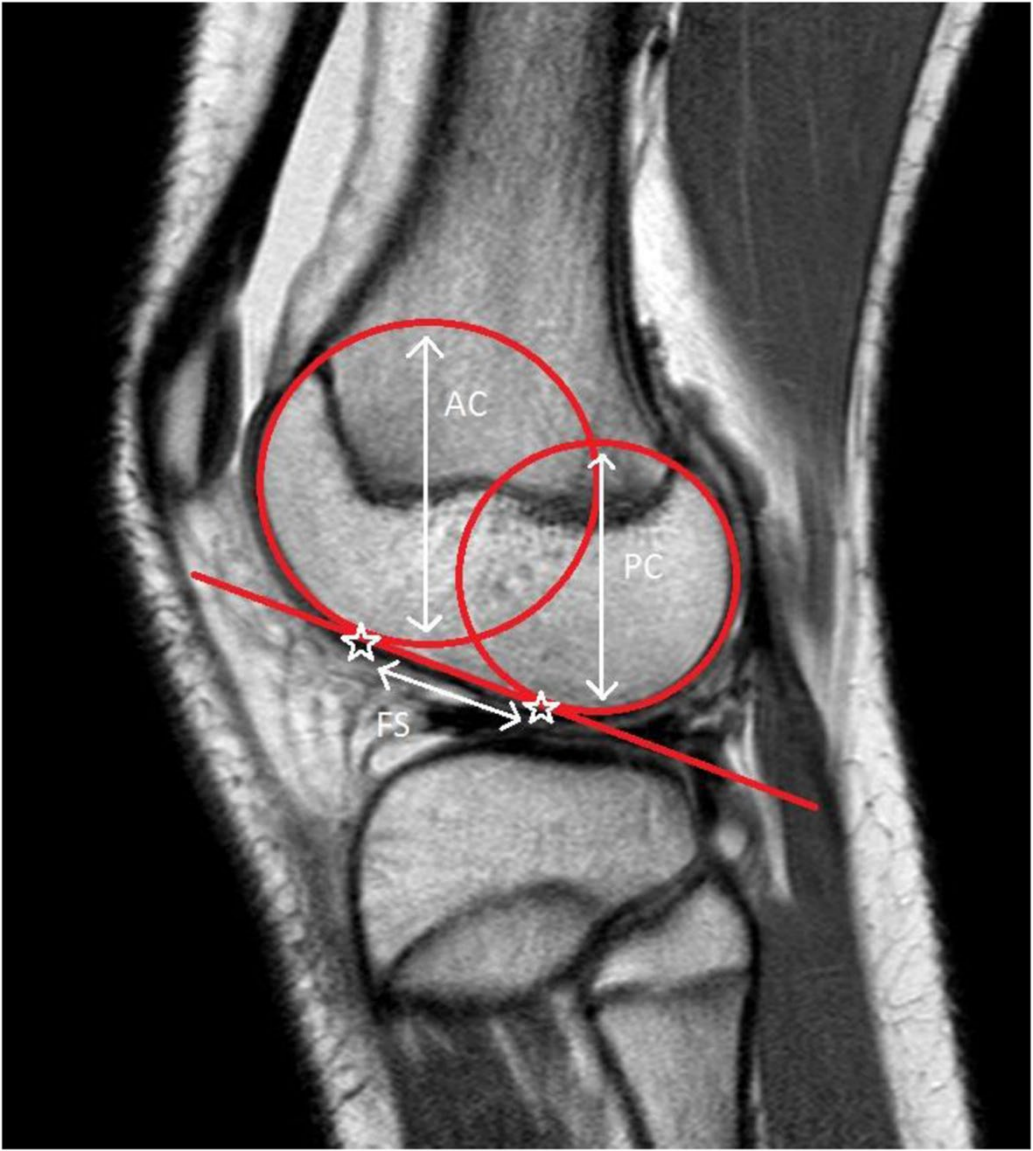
MRI measurements of the femur. The anterior “extension” circle [AC] and posterior “flexion” circle [PC] are drawn [14]. A line tangent to both circles determines the flat surface [FS] [31].
